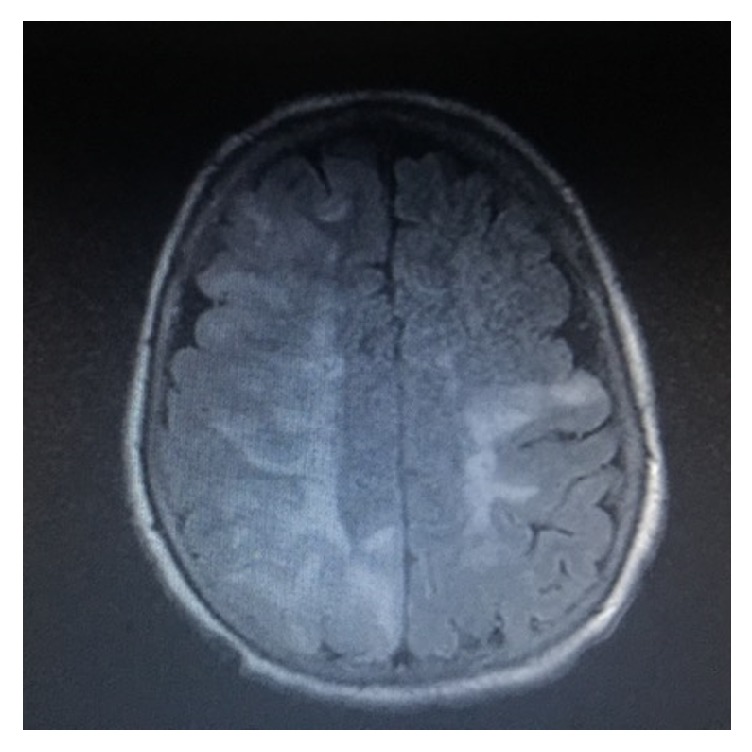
Initial MRI brain (FLAIR) showing bilateral frontal hyperintense white matter lesions, right > left.
